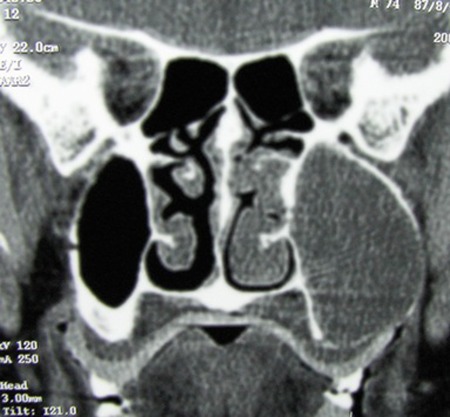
Coronal CT scan view shows a large expansile tumoral lesion located in left maxillary sinus, displacing medial and lateral walls.
